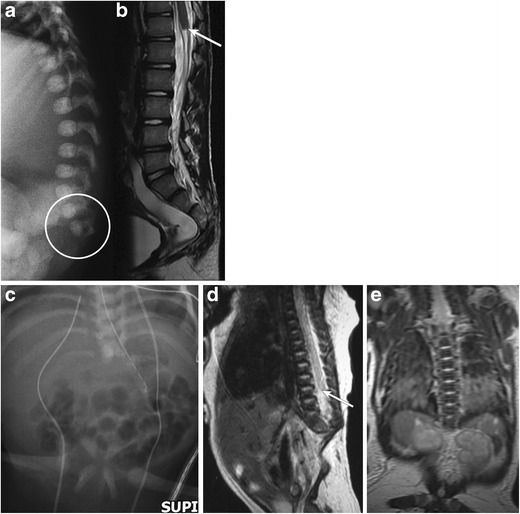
a-b: Caudal/sacral agenesis: Lateral radiograph of the spine (*a*) in one infant reveals absence of the distal scacrococcygeal segments. Accompanying sagittal T2-weighted MR image (*b*) of the lumbar spine reveals abnormal truncation of the conus medullaris (*arrow*). c-e: Caudal agenesis (another neonate): Frontal radiograph of the abdomen (*c*) in a neonate reveals absence of the entire lumbar and sacrococcygeal spine; the iliac bones are dysmorphic and abnormally close, though separate. Accompanying sagittal (*d*) and coronal (*e*) MR images of the spine reveal absence of the lumbar-sacrococcygeal segments of the spine. Abnormal high termination and truncation of the spinal cord is noted (*arrow* on *d*)
